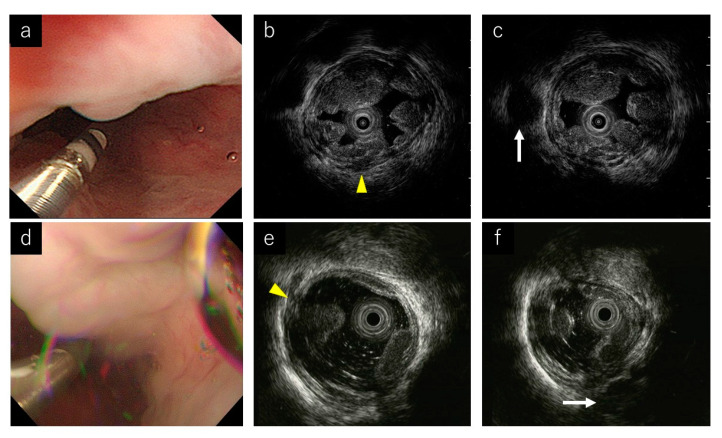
Endoscopic ultrasound (EUS) images of representative cases. (a) Endoscopic image during the jelly-filling method. (b,c) EUS images of the jelly-filling method. Yellow arrow head shows a perforating vein (Pv). White arrow shows a para-esophageal vein (Para-v). (d) Endoscopic image during the water-filling method. (e,f) EUS images of the water-filling method. Yellow arrow head shows a perforating vein (Pv). White arrow shows a para-esophageal vein (Para-v).
